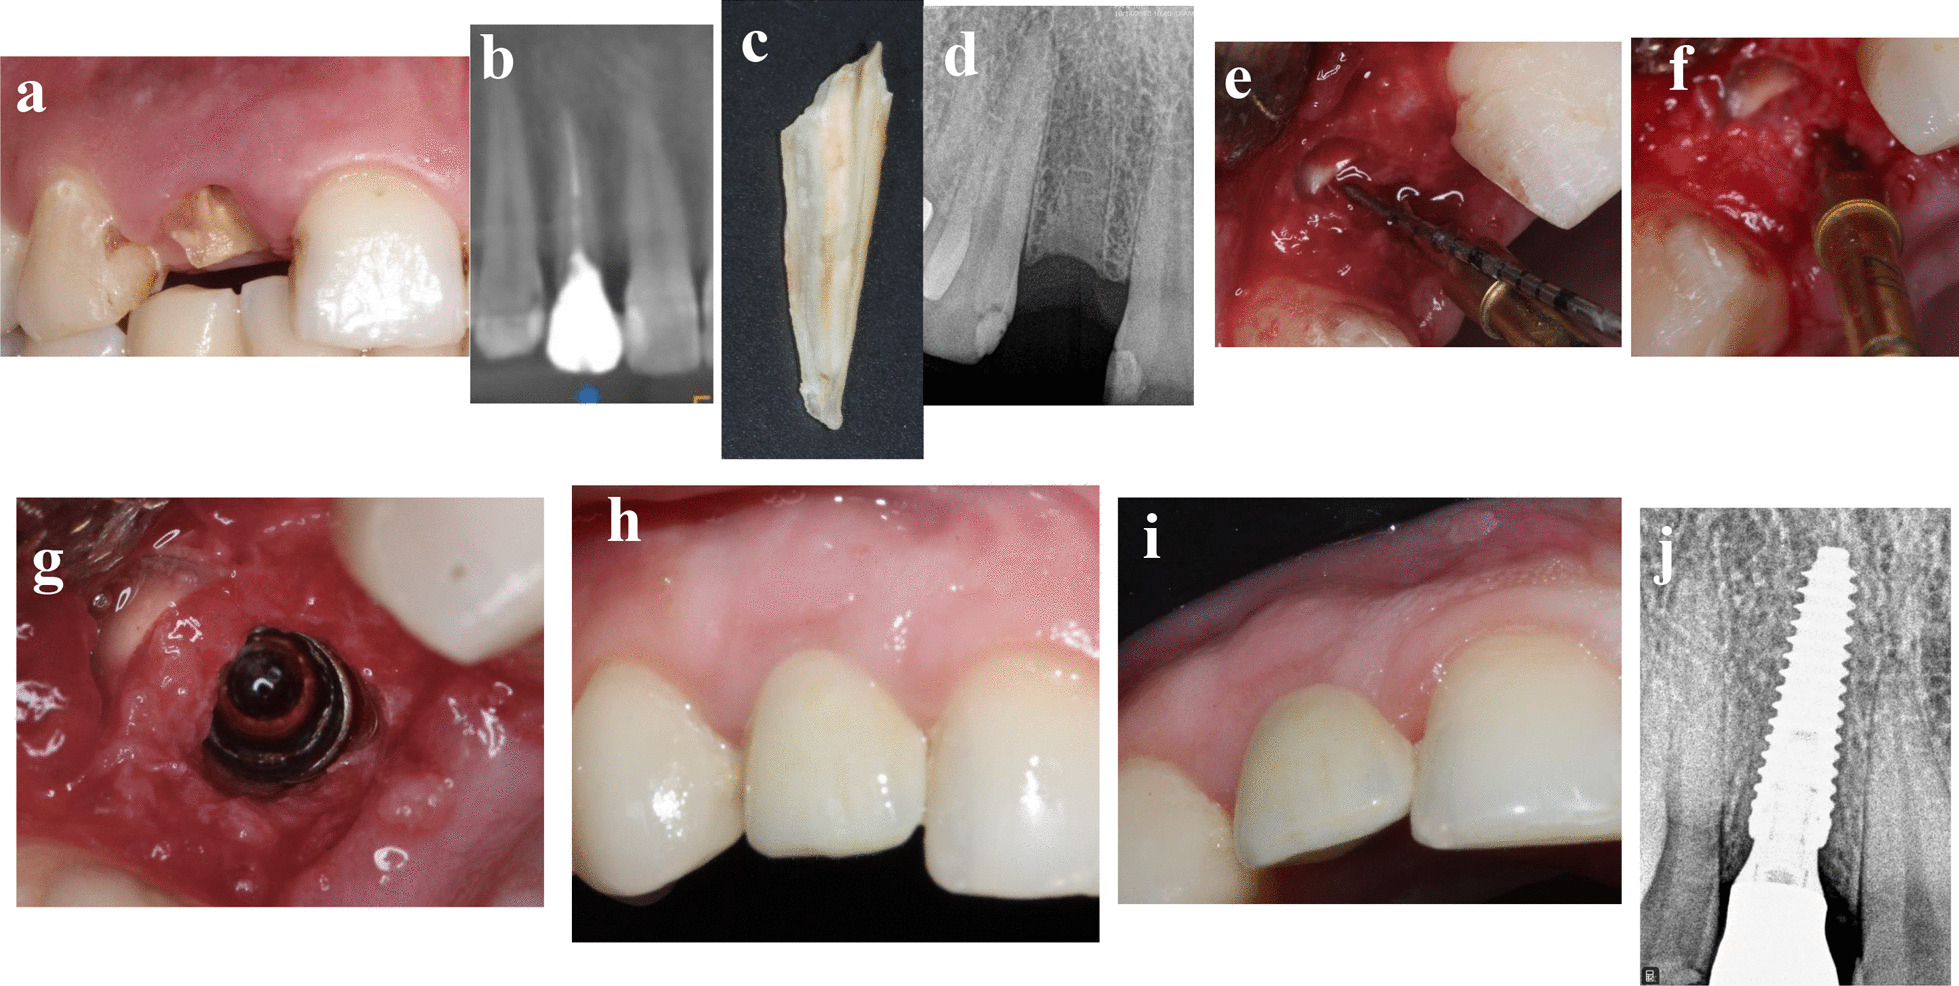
a–j Photos and radiographs of case #4 replacing maxillary right lateral incisor. a Preoperative frontal view; b panoramic view of preoperative CBCT; c the extracted palatal portion; d periapical radiograph after shield preparation; e the depth of soft tissue penetration measured with periodontal probe; f osteotomy preparation in healed socket palatal to the shield; g implant placed palatal to the shield; h frontal view of the of the final implant restoration at 5 months post-loading; i incisal view of the of the final implant restoration at 5 months post-loading; j periapical radiograph at 5 months post-loading
